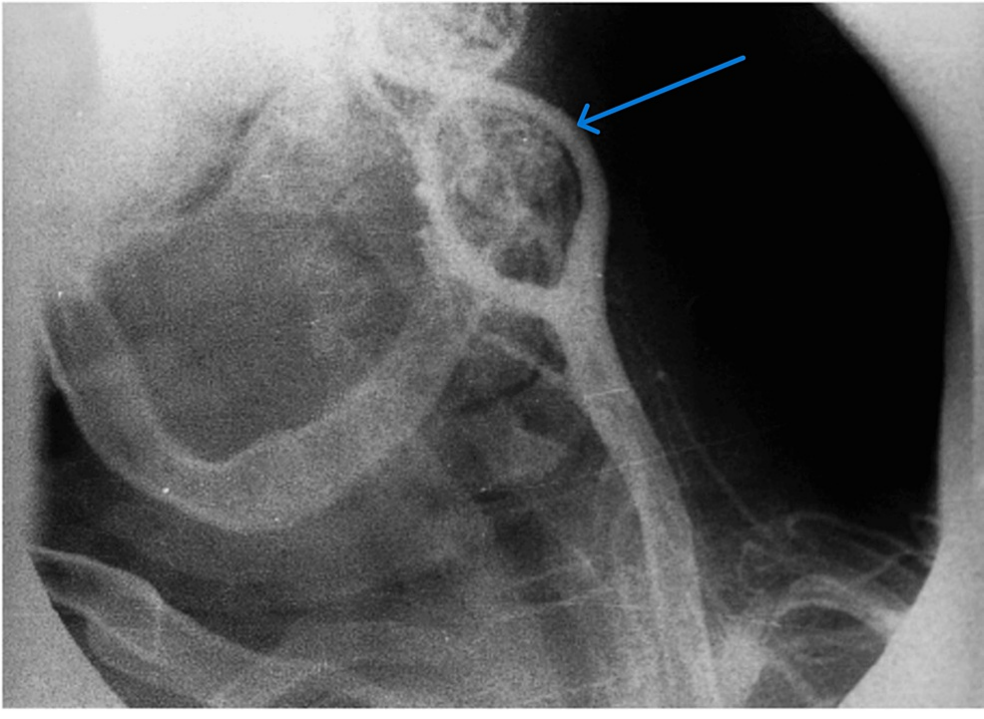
CT angiography of a carotid body tumor (lyre sign) case
